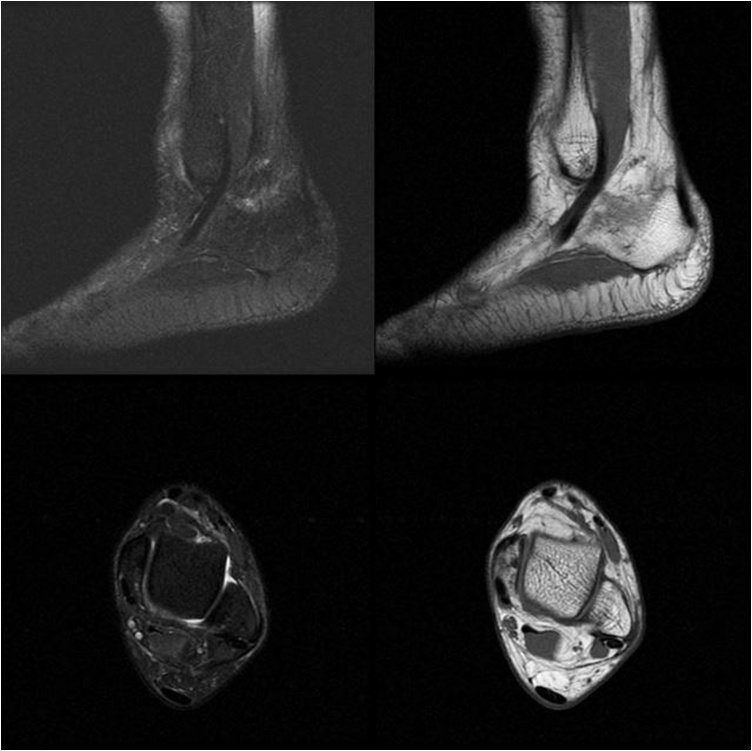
MRI showing peroneal tendon subluxation.
